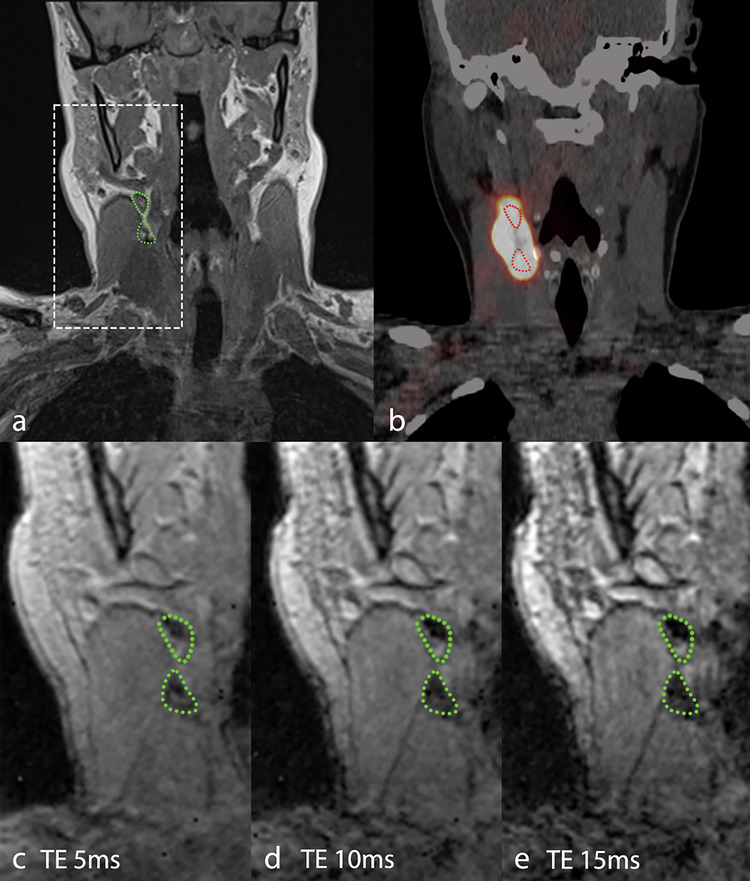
Coronal images of patient #10 with a right-sided lateralized tongue tumor after injection of 0.036 mL of SPIO. a T1-weighted VIBE Dixon in-phase image; b fused SPECT/CT; T2*-weighted images with computed TE of 5 ms (c), 10 ms (d), and 15 ms (e). The pathological nodal stage was pN0. Two lymph nodes are indicated by the green (MRI) and red (SPECT/CT) dotted line; the SPIO-induced susceptibility artifact is more prominent at longer echo times. MRI, Magnetic resonance imaging; SPECT/CT, Single-photon emission computed tomography/computed tomography; SPIO, Small particle iron oxide; TE, Echo time; VIBE, Volume interpolated gradient-echo
